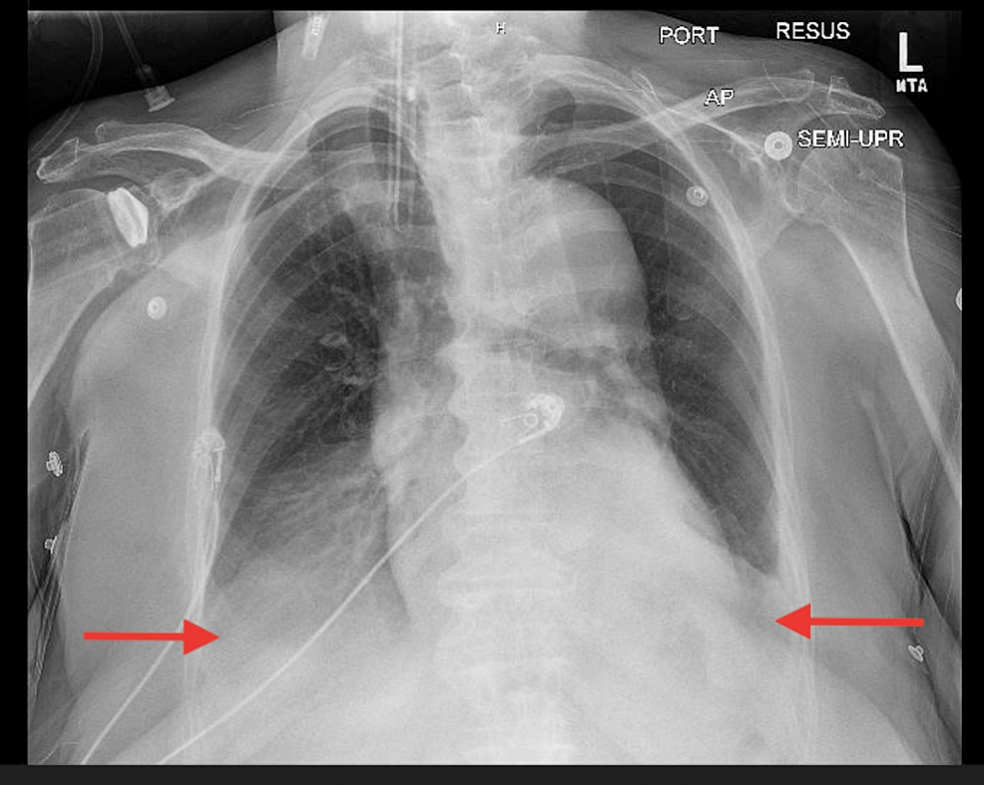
Chest X-ray revealing small bilateral effusions
